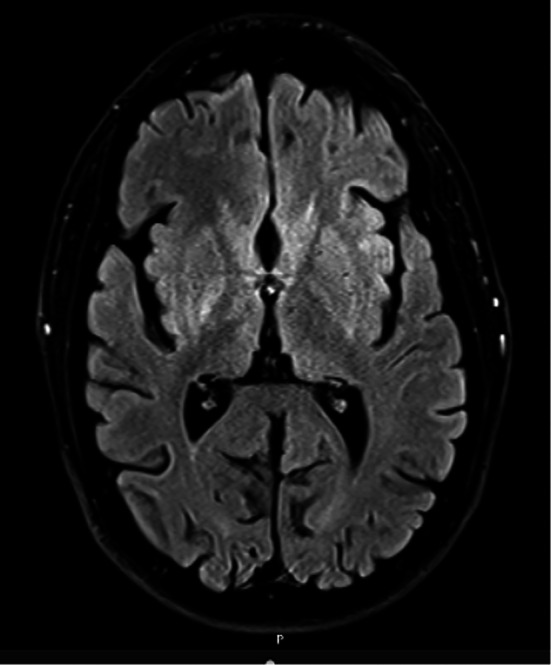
Axial T2‐FLAIR magnetic resonance imaging (MRI) of the brain showing asymmetric hyperintensity of the lentiform nuclei and thalamus. Additional sequences are available for review in the Supporting Information.
